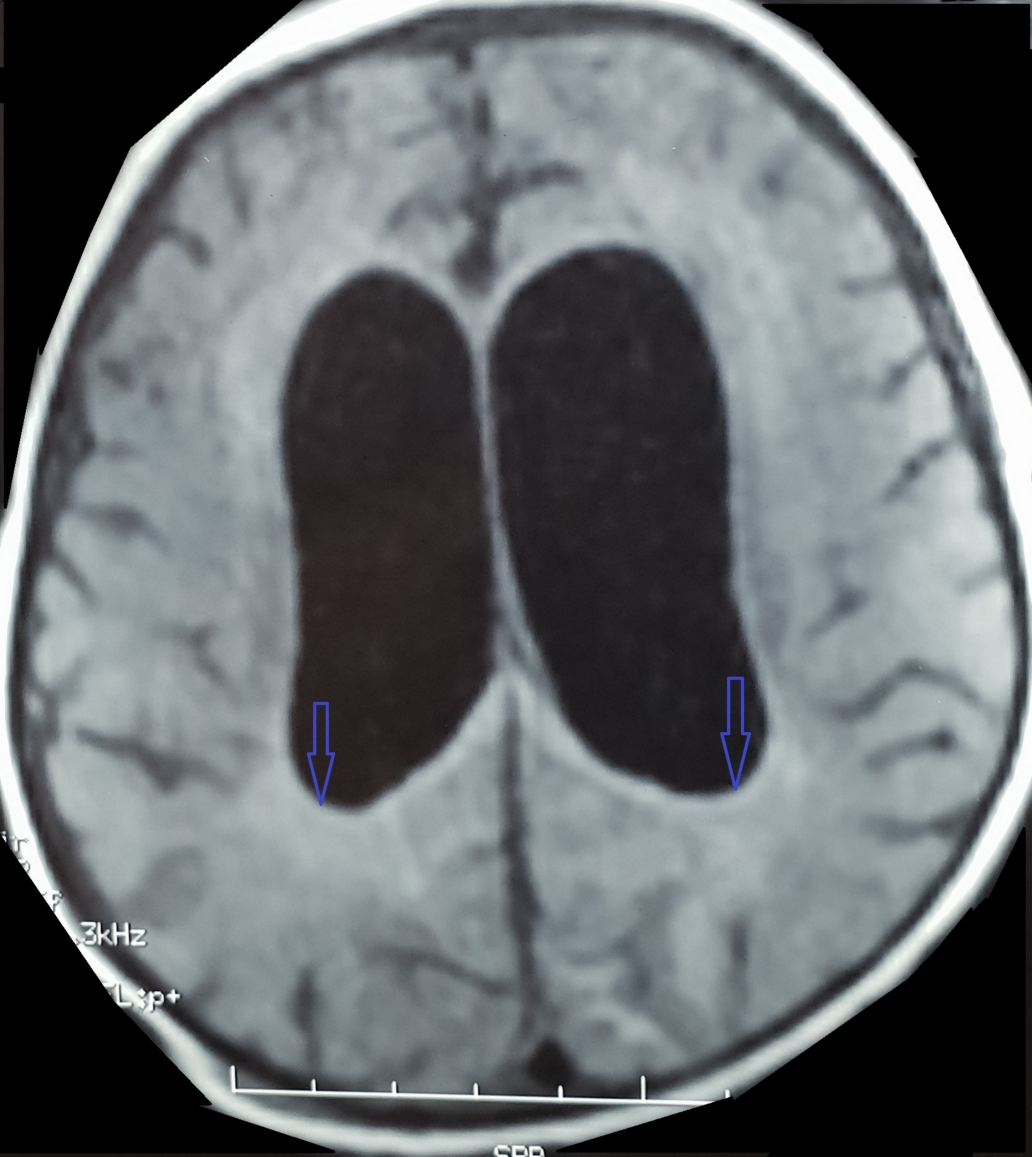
Axial cerebral MRI with FLAIR sequence showing ventriculitis.
